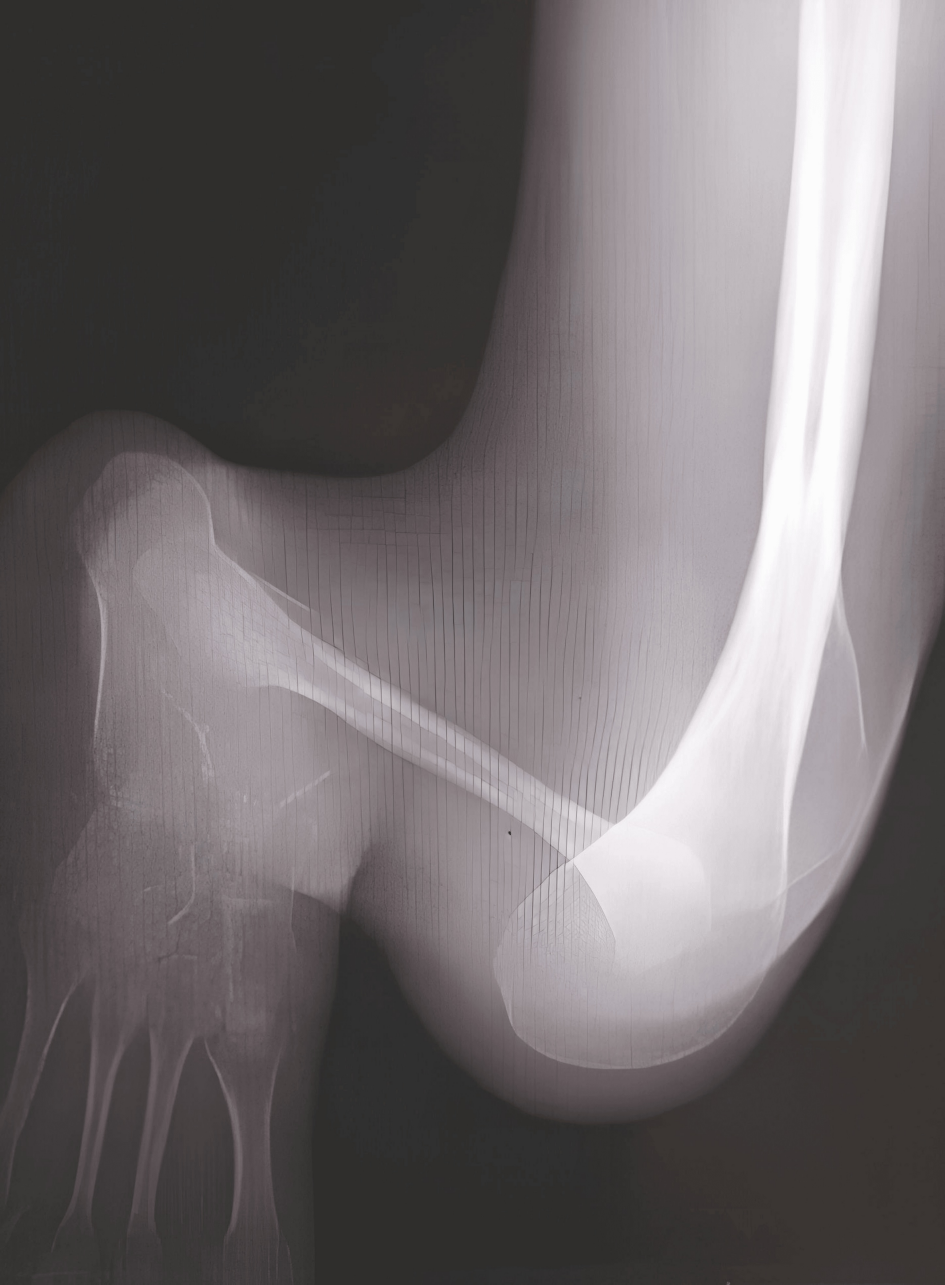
Lateral radiograph of the right lower limb demonstrating abnormal distal femoral morphology and absence of the tibia, characteristic of GWC GWC: Gollop-Wolfgang complex
